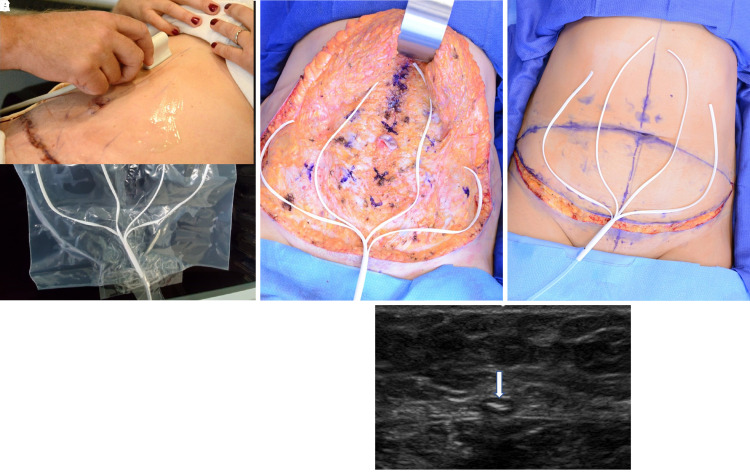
(A) As an example of manifold branch maintenance, fully opened manifold branches were placed inside a 5-gallon zip-lock bag and placed on Interi (Internal Closure System, IC Surgical, Grand Rapids, MI) suction. The branches remained in position while on suction. (B and C) Markings of manifold position were transposed to the overlying skin and followed for position maintenance postoperatively in this 32-year-old female. (D) High-resolution ultrasound (HRUS) confirming manifold position in its original location until device removal as shown in this 36-year-old female. (E) Cross-section HRUS image of a manifold laying on the abdominal fascia with no associated surrounding fluid.
